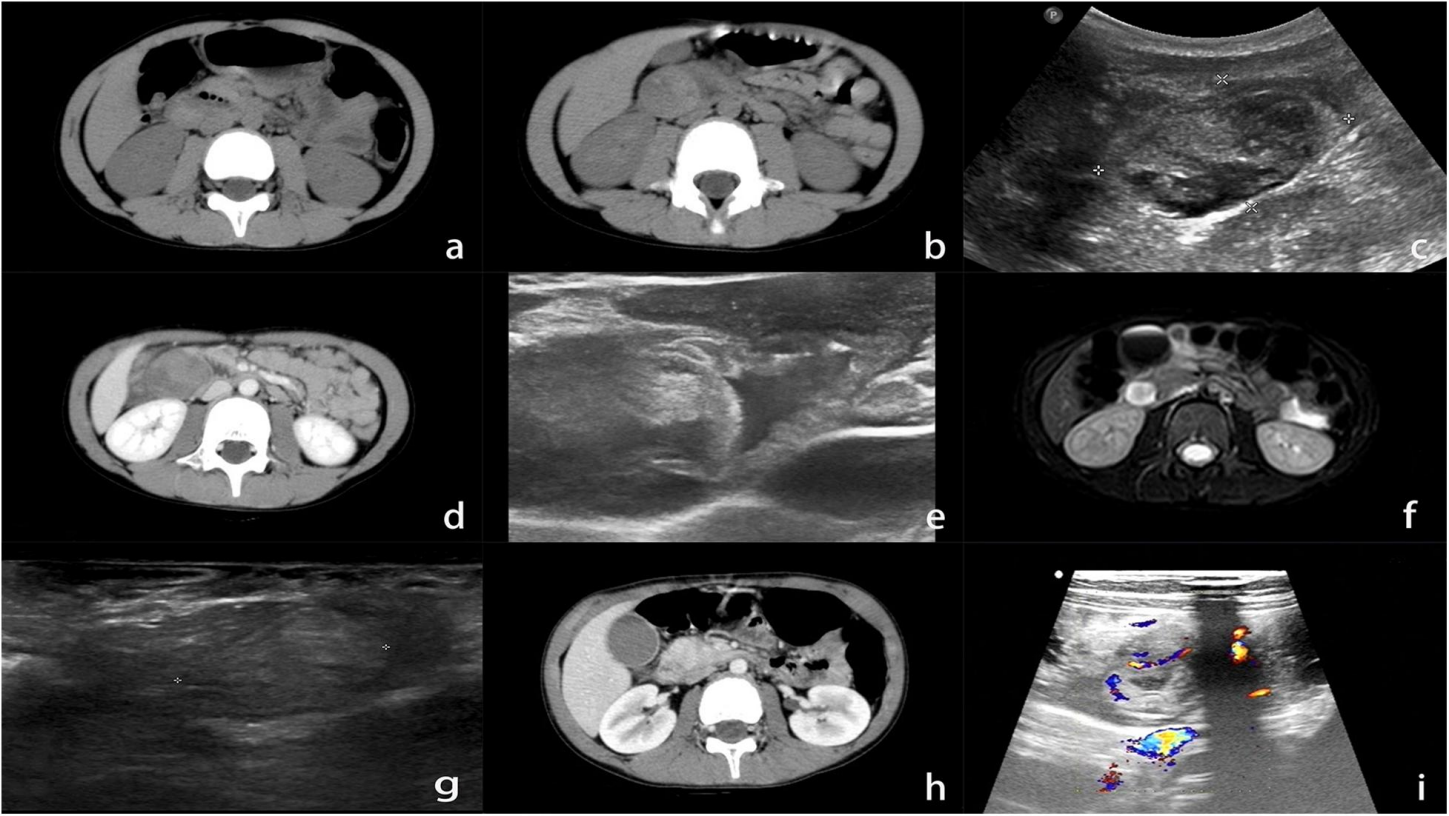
Imaging examination of duodenal wall hematoma in a child with car accident injury. (a) About 30 min after the car accident injury, the abdominal computed tomography (CT) scan showed no abnormalities. (b) After 10 h, abdominal pain worsened. Re-examination by abdominal CT showed a circular mass shadow in the descending part of the duodenum with uneven density. (c) After admission, upper abdominal ultrasound showed a circular heterogeneous echo in the descending part of the duodenum and intestinal wall. (d) On the second day of conservative treatment, a CT scan showed that the hematoma in the descending part of the duodenum was slightly larger than before, with uneven density and locally higher density. No enhancement was observed after administration of contrast media. (e) On the 5th day of conservative treatment, high-frequency ultrasound showed that the hematoma had reduced in size, accompanied by cystic echoes inside, protruding towards the intestinal cavity. (f) On the 10th day of conservative treatment, magnetic resonance imaging re-examination showed a hematoma in the descending wall of the duodenum, presenting as a short TI and long T2 signal shadow. (g) On the 12th day of conservative treatment, high-frequency ultrasound showed hematoma shrinkage and low echogenicity. (h) On the 15th day of conservative treatment, an abdominal CT scan showed a significant reduction in the hematoma in the descending part of the duodenum. (i) On the 20th day of conservative treatment, high-frequency ultrasound showed thickening of the duodenal wall, with hypoechoic hematoma inside. Color Doppler flow imaging showed no blood flow, while blood flow was visible in the surrounding intestinal wall.
